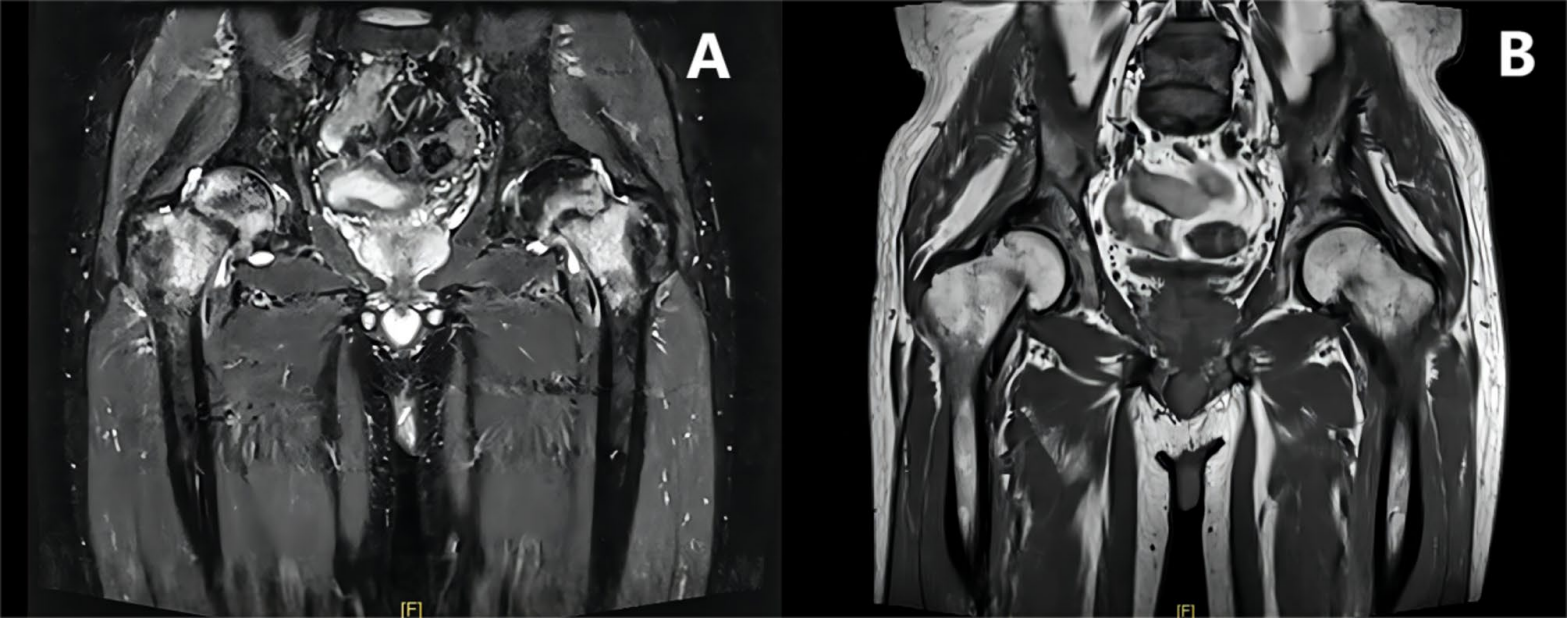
MR images of the patient’s hip joints. A Bilateral fractures of the femoral neck occurred in June 2015. B Most of the bilateral femoral neck fractures healed in September 2017
